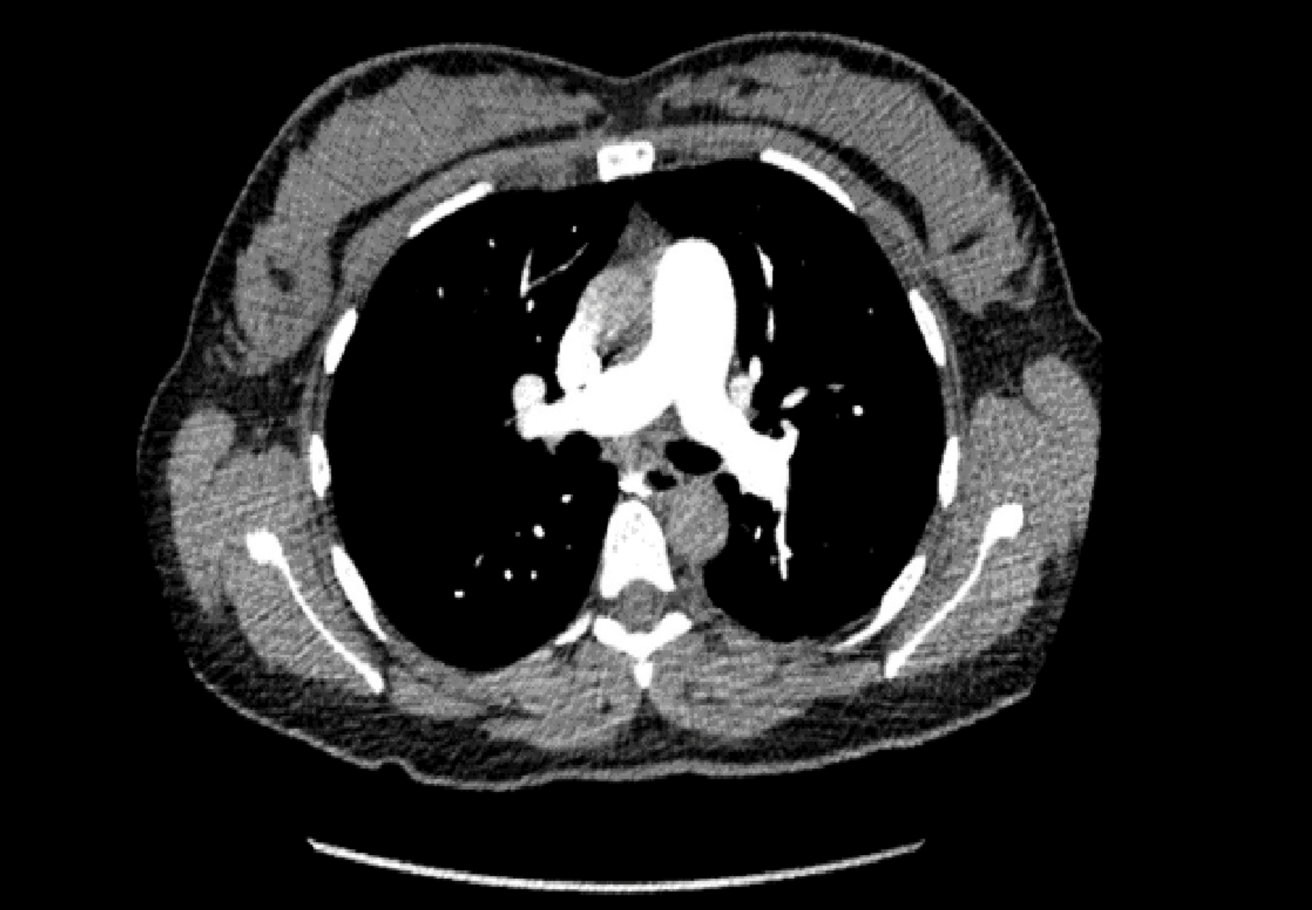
CT pulmonary angiography showing the absence of any evidence of pulmonary embolism.
